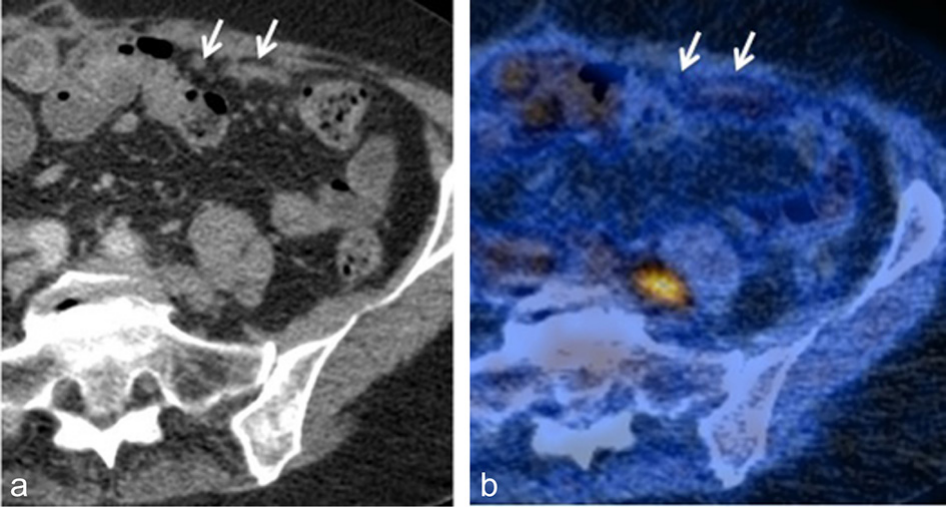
A 72-year-old female with serous carcinoma of the ovary. (a, b) Demonstration of multiple small histo-pathologically confirmed sub centimetre anterior peritoneal nodules not FDG avid but detected on the CT component of ^18^F-FDG PET CT.
